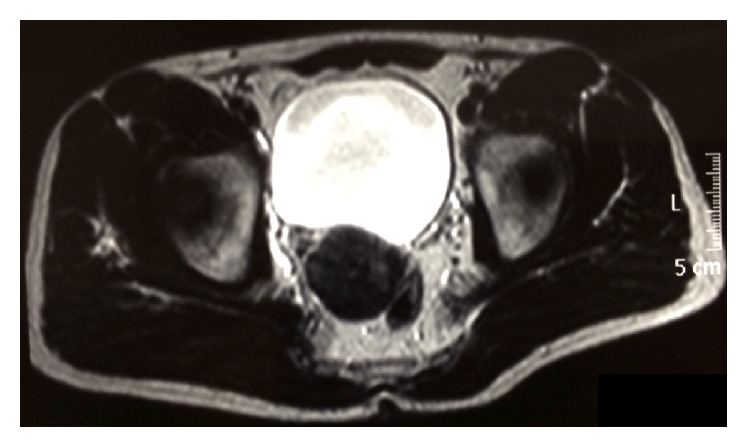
Magnetic resonance imaging T2-weighted imaging showed a mass of 5 cm in diameter on the posterior wall of the bladder.
